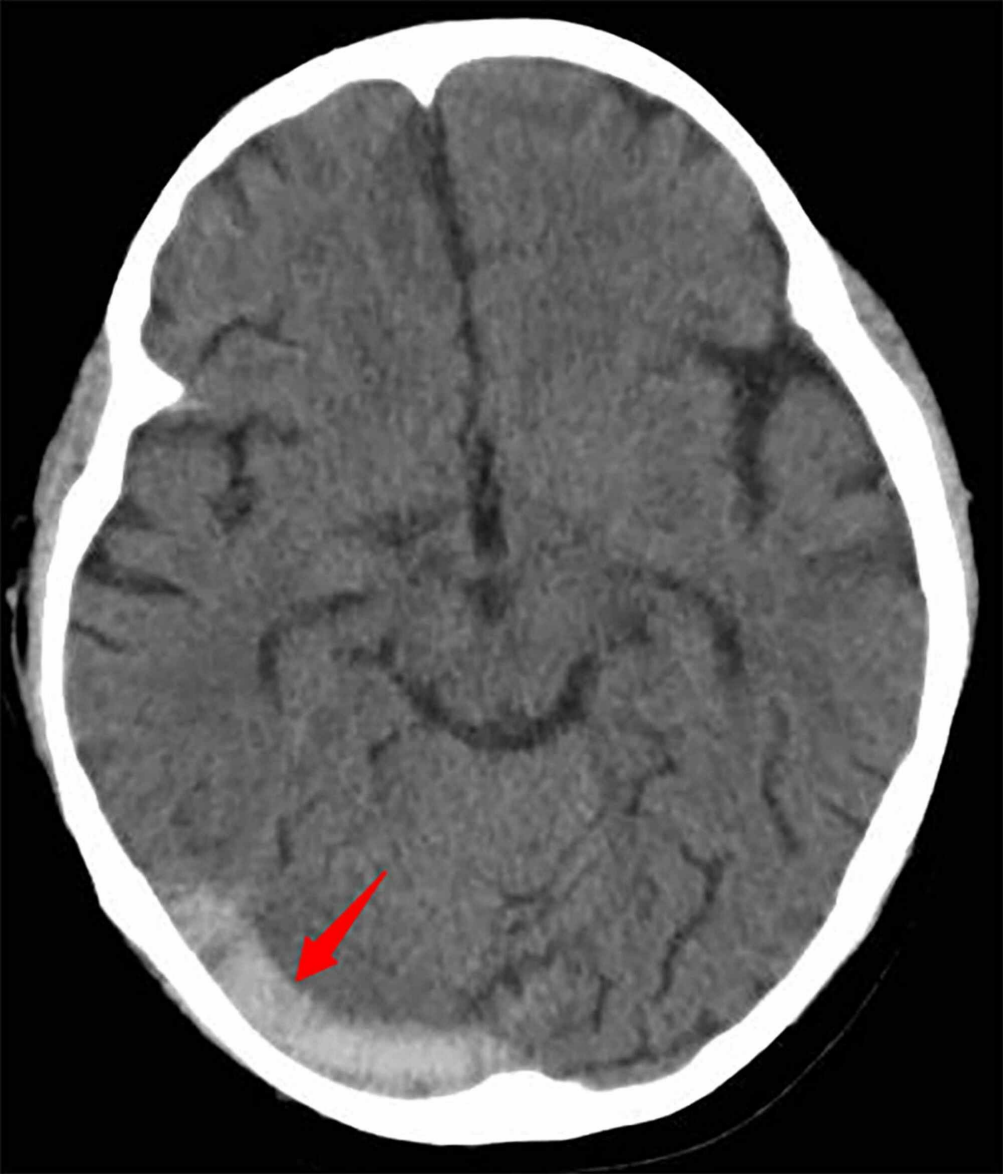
Brain CT On day five, brain CT shows the right sigmoid and the transverse sinuses with high density as shown by the red arrows.
